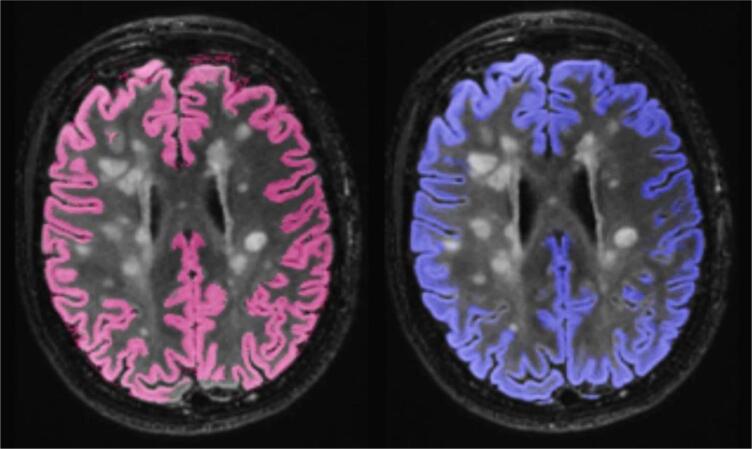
GM segmentation performance in the context of high WM lesion load, using the new GIF database (pink = T1, blue = FLAIR).
